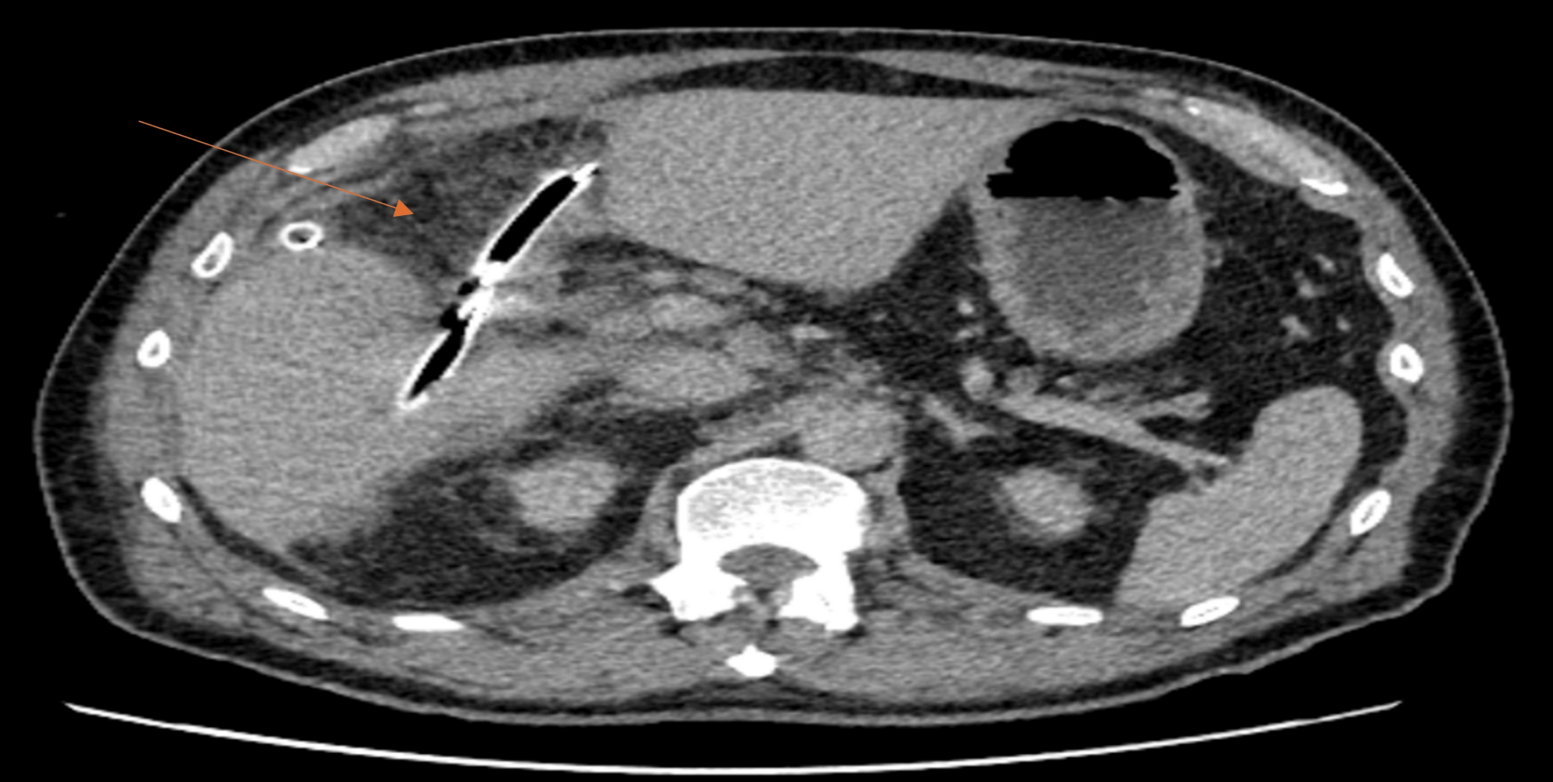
CT showing subscapular collection CT abdomen demonstrating enlarging subcapsular collections in segments VI-VII with intralesional gas, suggestive of secondary infection.
